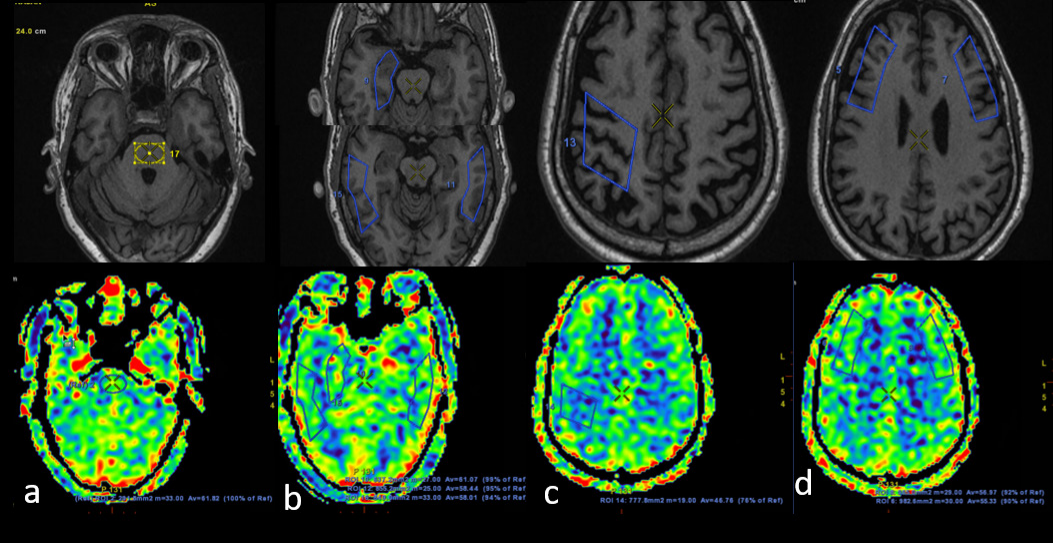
In the same patient, manual ROI was drawn on the ASL image (lower row), which showed significant FDG-PET hypometabolism, taking pons (a) as a reference (upper row shows T1W image for an anatomical landmark). ROIs and their relative percentage of perfusion are shown for the left occipital (b), bilateral lateral frontal, left parietal (c), and the left medial frontal region
